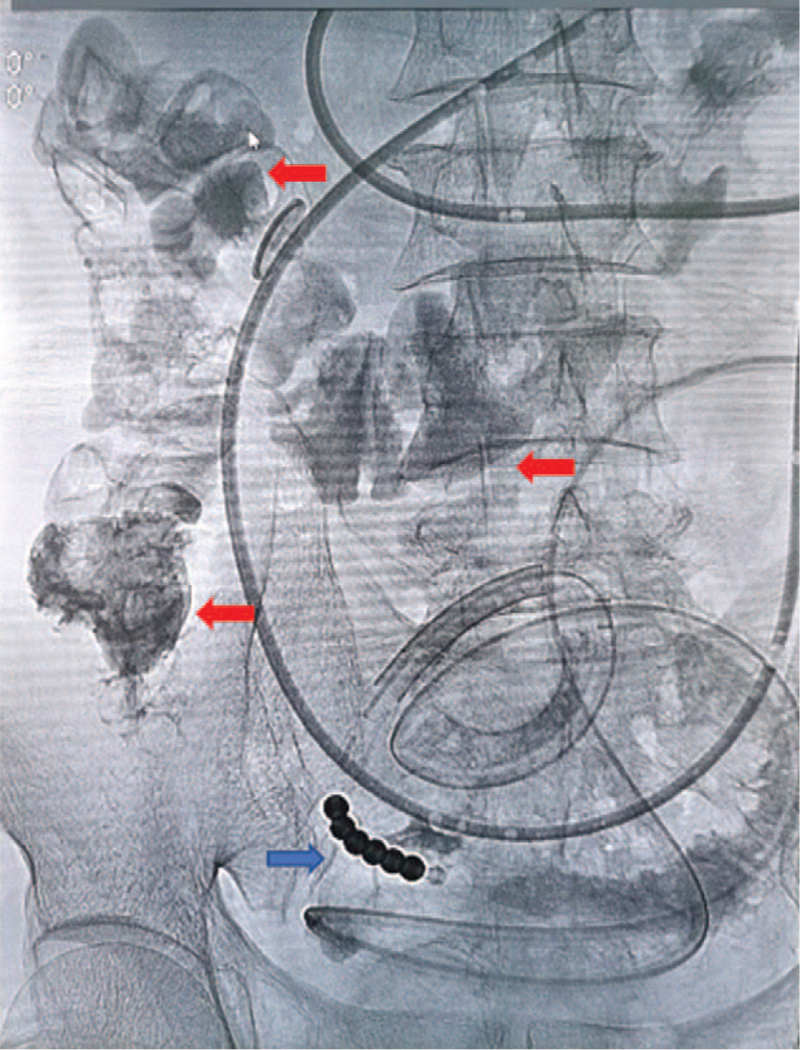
Abdominal X-ray showed the position of the ileus tube (blue arrow indicates the end of the ileus tube, red arrows indicate the visible colon).
